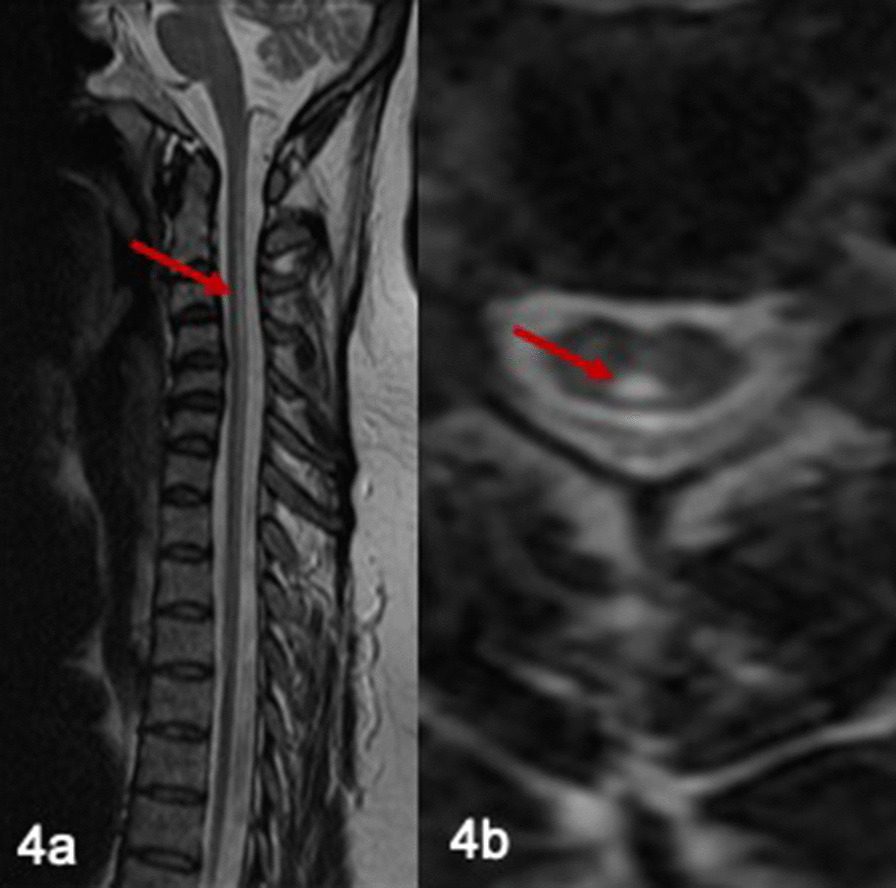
Spinal MRI in patient 3. MRI spine showed a focus of high signal in midline posteriorly extending from cranio-cervical junction to level T5-6 (a, b)
